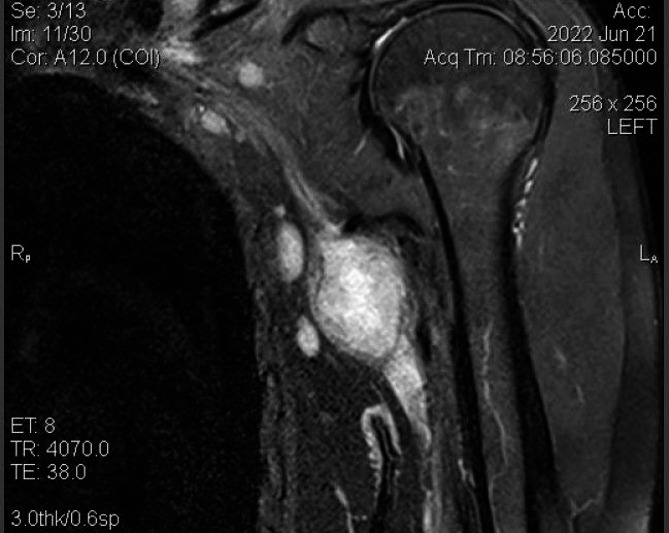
Magnetic resonance imaging (MRI) of the axillary mass
